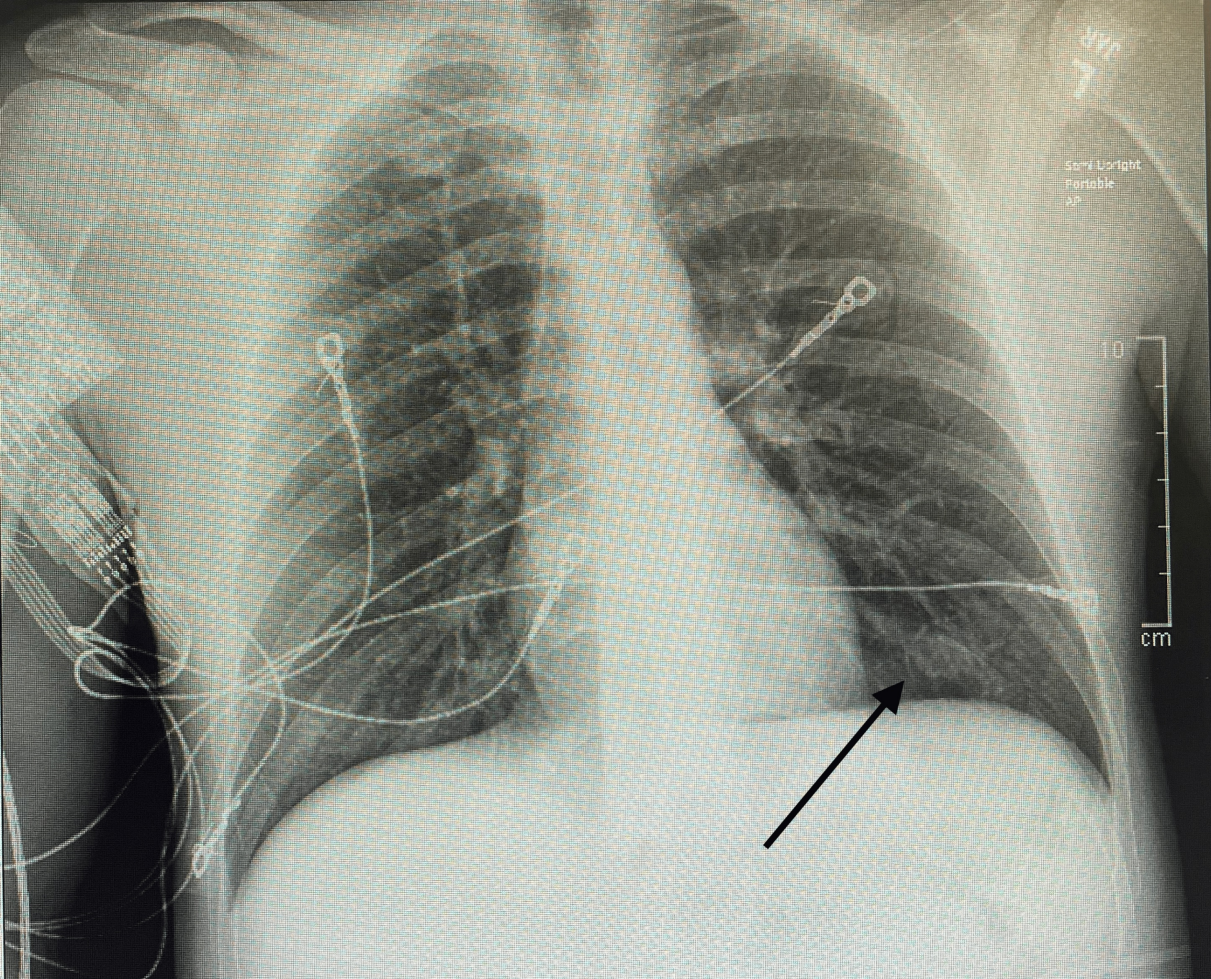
Portable anteroposterior chest radiograph demonstrating clear lung fields without evidence of consolidation, effusion, or pneumothorax. A black arrow highlights the representative normal left lower lobe region.
